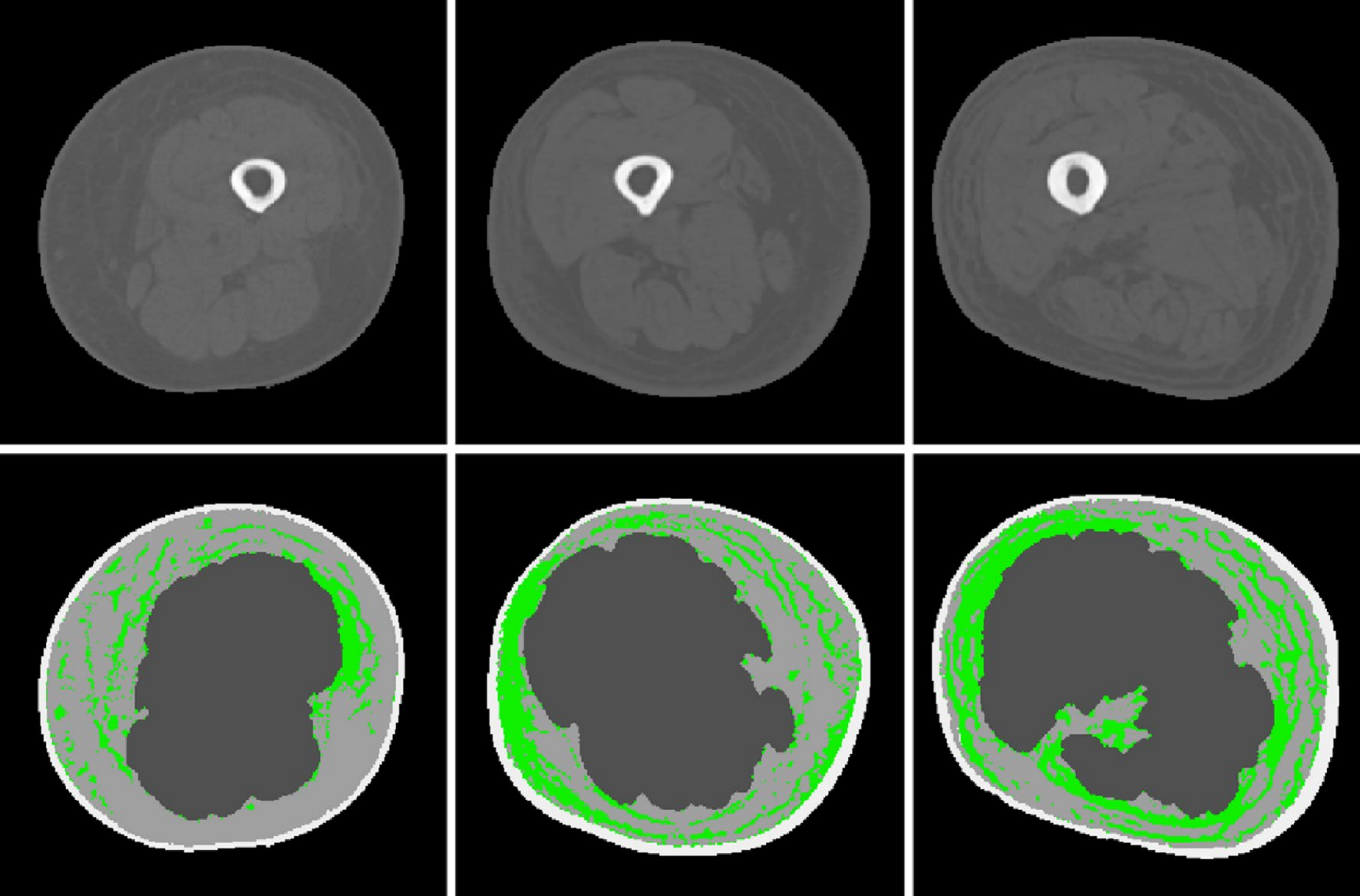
Original CT images (upper row) and images labeled using the lab-developed GUI (lower row). The pixels classified as fibrosis are marked in green.
